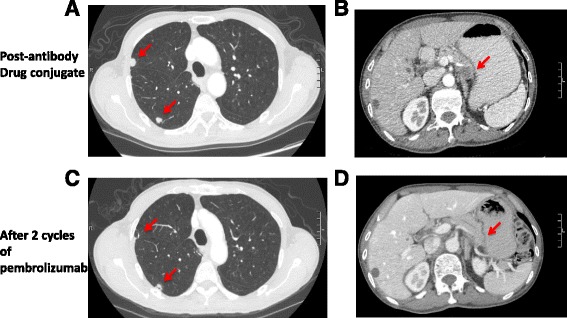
CT findings. a CT chest after 1 cycle of the antibody drug conjugate with new pulmonary nodules. b CT abdomen after 1 cycle of an experimental antibody drug conjugate against a neuroendocrine cell surface marker with a new pancreatic lesion. c Improvement in lung nodules after 2 cycles of pembrolizumab. d Resolution of the pancreatic lesion after 2 cycles of pembrolizumab
